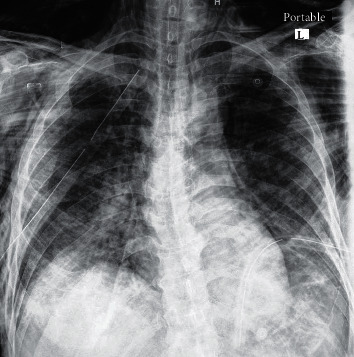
Chest X-ray showing pneumomediastinum and subcutaneous emphysema with inserted thoracostomy tube.
